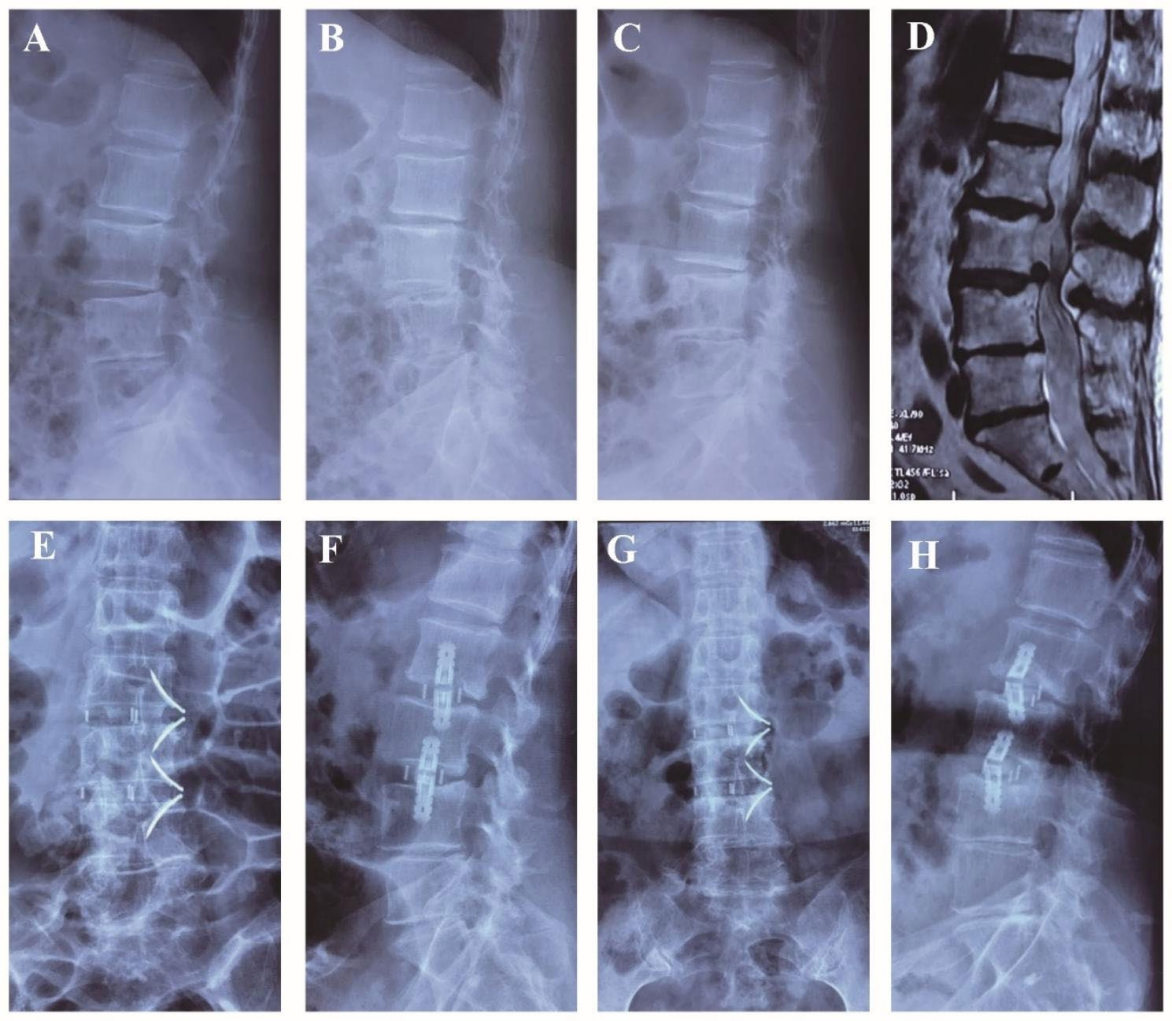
Pre-, Post-operative and follow-up images obtained in a 76-year-old female who had low back pain with radiating pain in the lower extremity, exacerbated during exercise and presented with L2-4 instability. The symptoms disappeared after SA-LLIF. (A) The lateral X-ray shows lumbar degenerated spondylolisthesis; (B, C) The flexion-extension lateral X-rays shows L2-4 instability; (D) MRI fat-suppressed image shows L3-5 intervertebral disc degeneration and herniation; (E, F) good lumbar alignment and satisfactory fixation were achieved after operation; (G, H) 3 months after operation, the AP and lateral X-ray shows the plate and cage were stable
